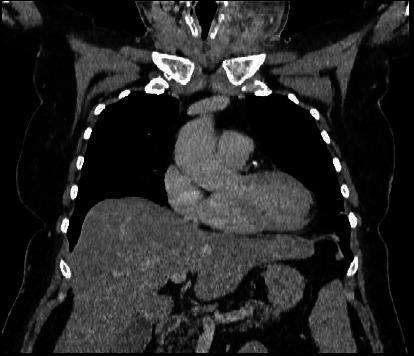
Initial CT of the chest.
